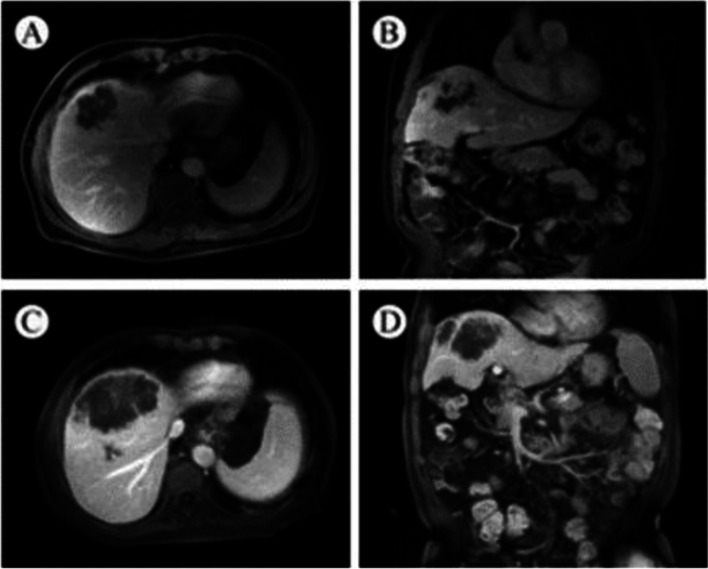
MRI showed pseudo-progression of the primary tumor. A and B MRI in January 2021. C and D MRI in July 2021. Abbreviations: MRI, magnetic resonance imaging
